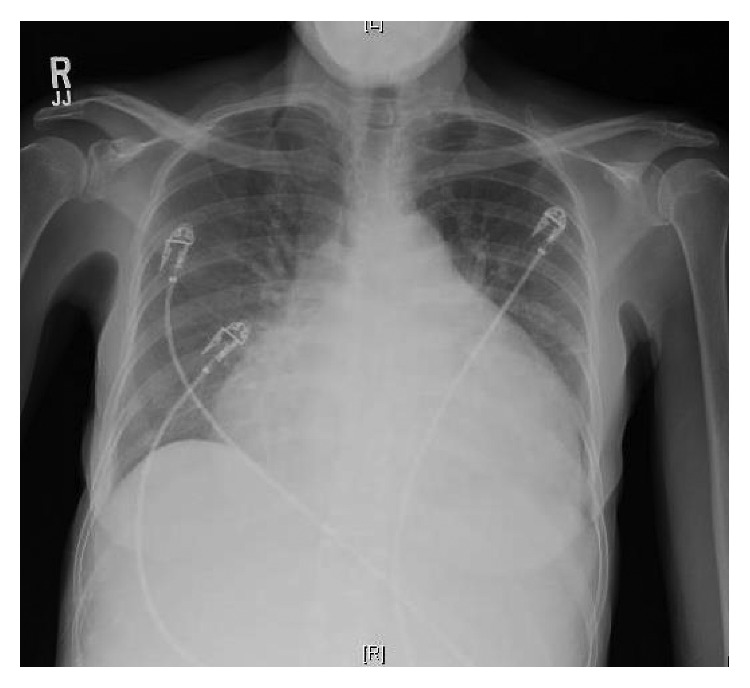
Chest X-ray of the patient on admission demonstrating markedly enlarged cardiac silhouette with “water-bottle” appearance.
